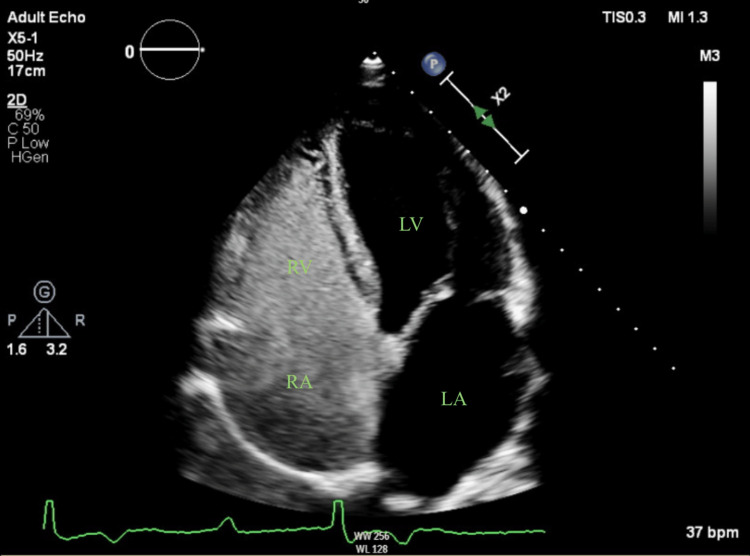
Apical four-chamber view after agitated saline injection showing no shunts RA, right atrium; RV, right ventricle; LA, left atrium; LV, left ventricle
